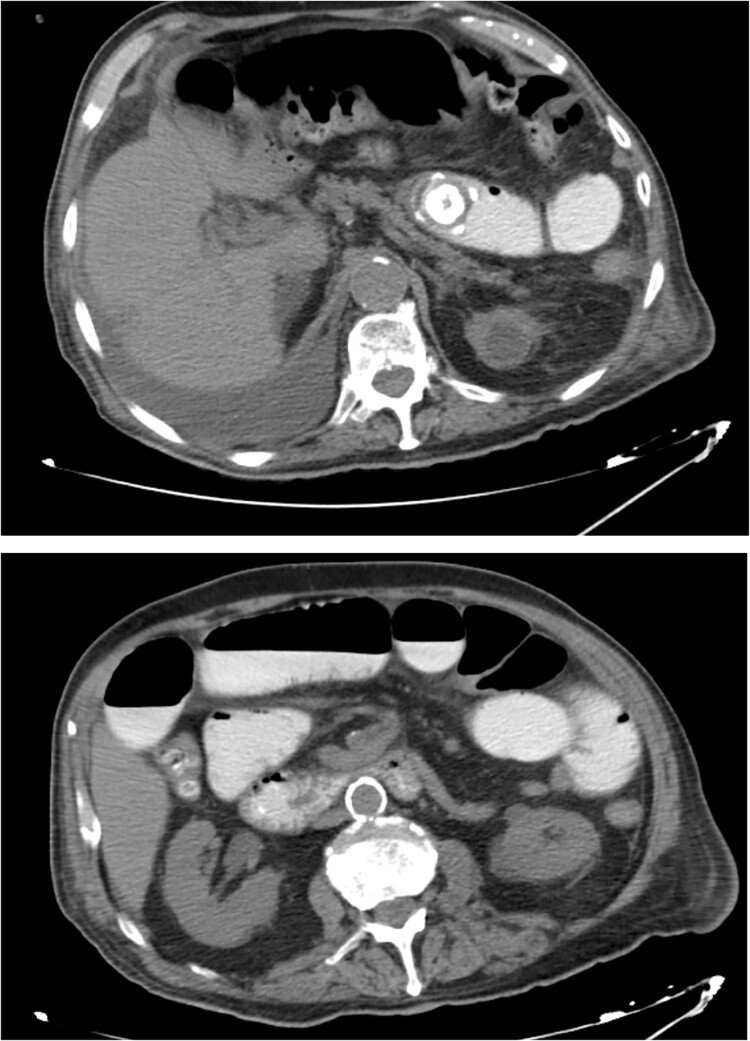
CT images of the abdomen revealed small bowel obstruction due to gallstone and presence of air and oral contrast into the gallbladder.
